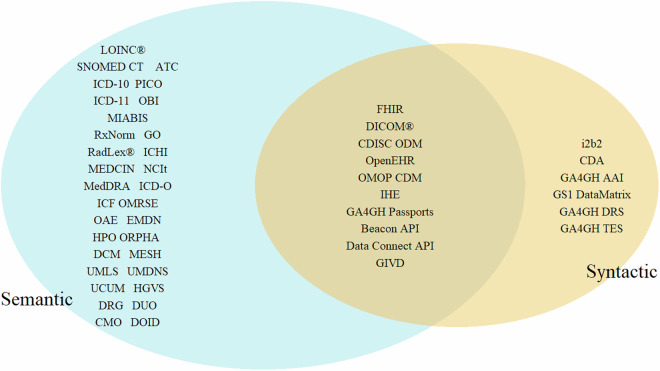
Identified syntactic and semantic standards in health research. We categorized health research and interoperability standards into three types: semantic, syntactic, or both. Semantic standards focus on the meaning and interpretation of data, including terminologies, vocabularies, and ontologies (e.g., SNOMED CT, LOINC, ICD). Syntactic standards focus on the structure and format of data exchange, defining how data is formatted and transmitted (e.g., HL7 CDA). Combined standards include elements of both, defining data structure and format while also ensuring consistent meaning with value sets or terminologies (e.g., HL7 FHIR).
